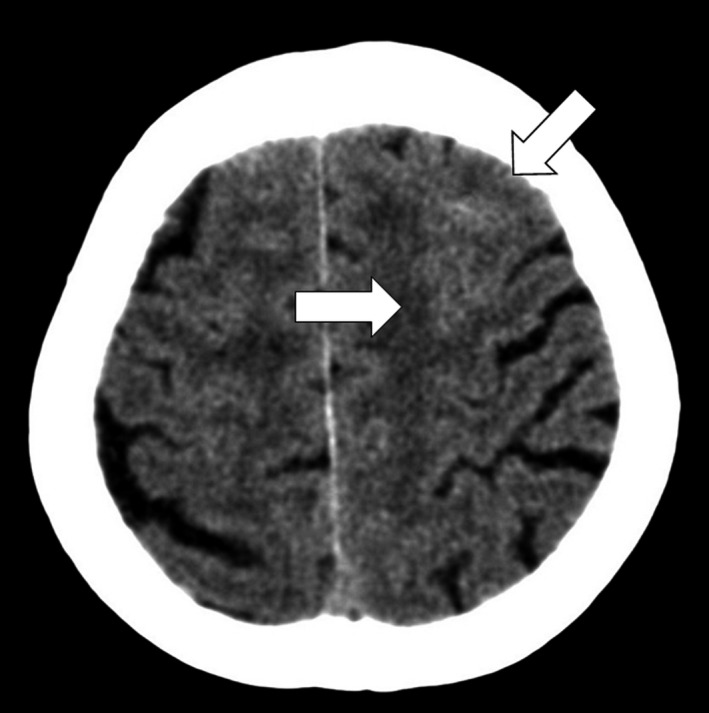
Head CT revealed high densities and obscurations in left high‐convexity frontal sulci (arrows)
